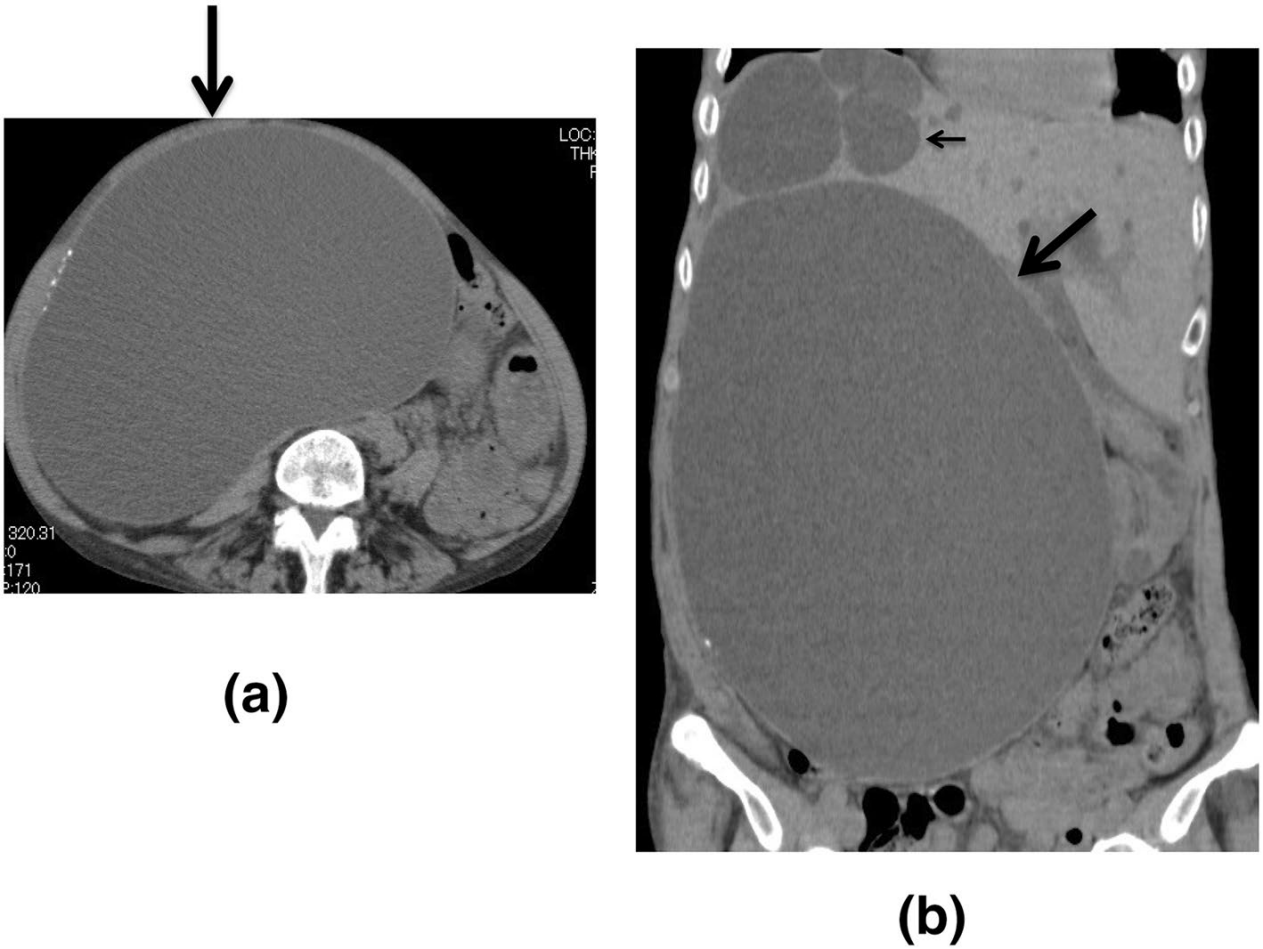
Computed tomography showed a huge cyst, and contracted kidneys with multiple small cysts. Laparoscopic fenestration surgery was performed for huge cyst measuring 26.1 cm × 23.7 cm × 16.1 cm (large arrow) and small cyst measuring 3.1 cm × 2.9 cm × 2.6 cm (small arrow). a Axial section, b coronal section
